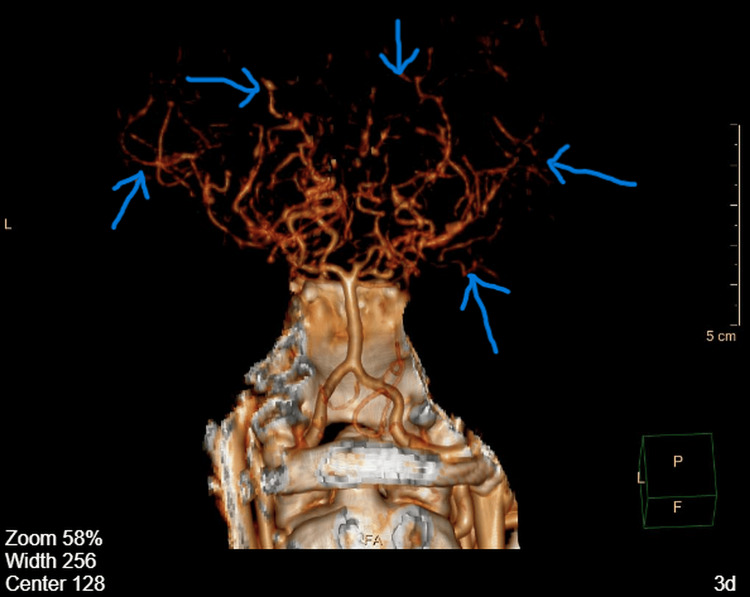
Three-dimensional CT angiography reconstruction demonstrating extensive intracranial collateral circulation in Moyamoya disease Three-dimensional CT angiography (CTA) reconstruction of the intracranial vasculature showing a dense network of abnormal collateral vessels in the bilateral cerebral hemispheres. This abnormal vascular network represents the characteristic collateral circulation seen in Moyamoya disease, formed as a compensatory response to progressive stenosis or occlusion of the intracranial internal carotid arteries and their proximal branches. Blue arrows indicate the abnormal collateral vessels forming the Moyamoya collateral network.
